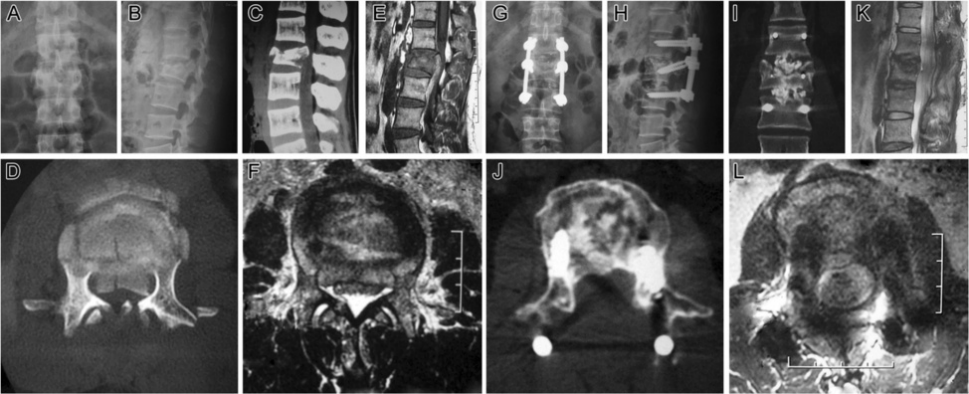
Follow-up images (case 18). Preoperative and postoperative imaging of a 35-year-old male patient (case 18) with L2 burst fracture, LSS _7, AO type A3.3, Danis type A with Frankel B neurologic impairment. A, B, Preoperative AP and lateral roentgenogram showing a L2 burst fracture with an angular segmental deformity of 21°. Preoperative sagittal (C) and axial (D) CT scan image, preoperative sagittal (E) and axial (F) lumbar MRI demonstrating a significant spinal canal encroachment at the fracture level. G, H, nine months postoperative AP and lateral plain radiograph showing a correct position of two cages placed to intervertebral space and the posterior transpedicular system. Angular deformity was corrected and segmental height was restored. I, 9 months postoperative coronal CT demonstrating successful intersomatic bony fusion of the L1 to L3 segment. J, 9 months postoperative axial CT demonstrating significant spinal canal clearance and remodeling of the posterior wall of the fractured vertebra body. K, L, 9 months postoperative sagittal and axial MRI showing satisfied decompression of the neural eliments.
